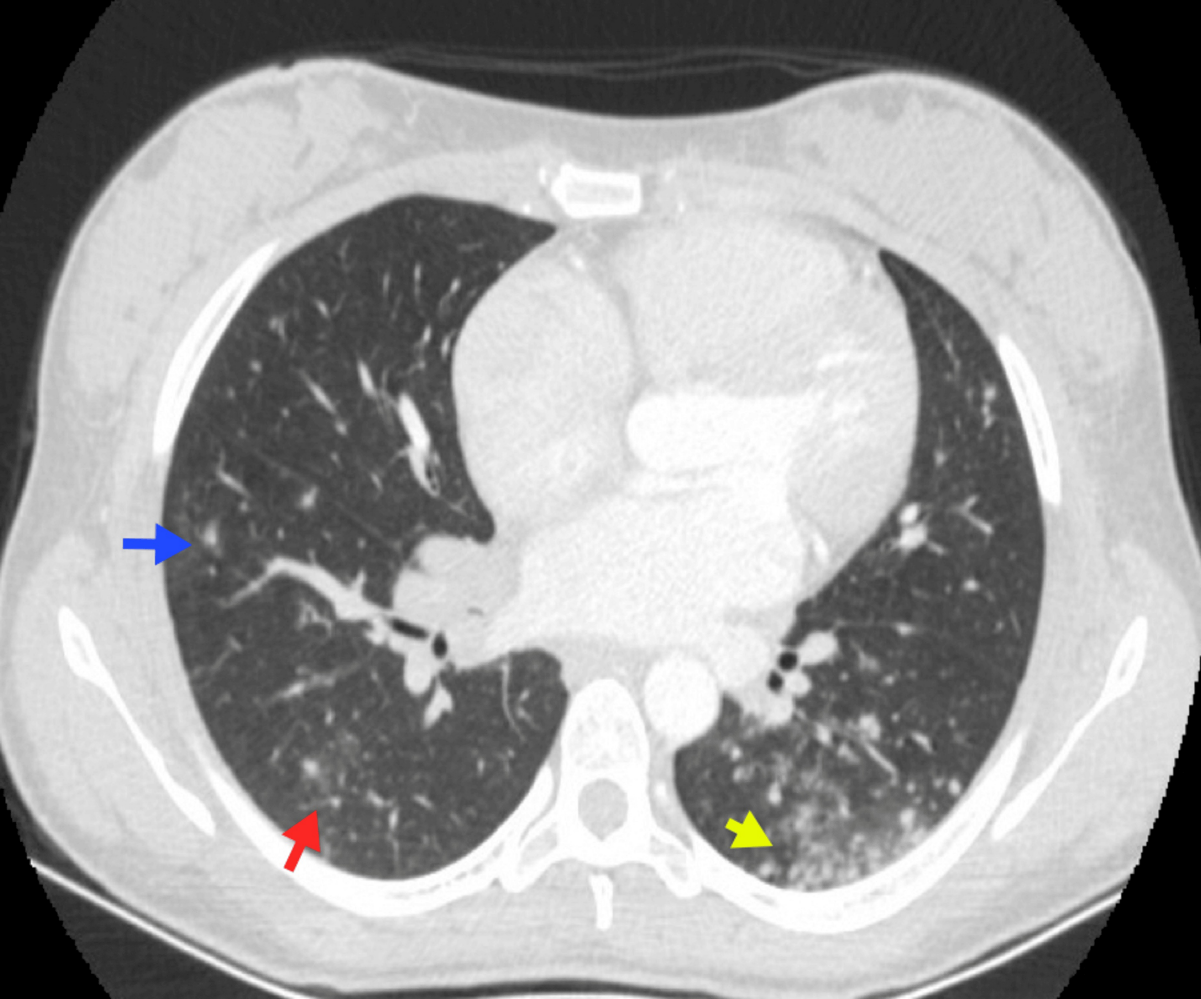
Fungal infection A 28-year-old female patient with nongerminal center diffuse large B-cell lymphoma and a prior history of cryptococcal meningitis. Chest CT reveals multiple centrilobular nodules in the lung parenchyma (blue arrow), ground-glass opacities in both lungs (red arrow), and basal consolidation in the left lung (yellow arrow). *Cryptococcus* spp. was isolated from the BAL. BAL, bronchoalveolar lavage Image courtesy: Pulmonology Service of the Internal Medicine Department, University of Antioquia. Consent for open access publication was obtained from the patient.
